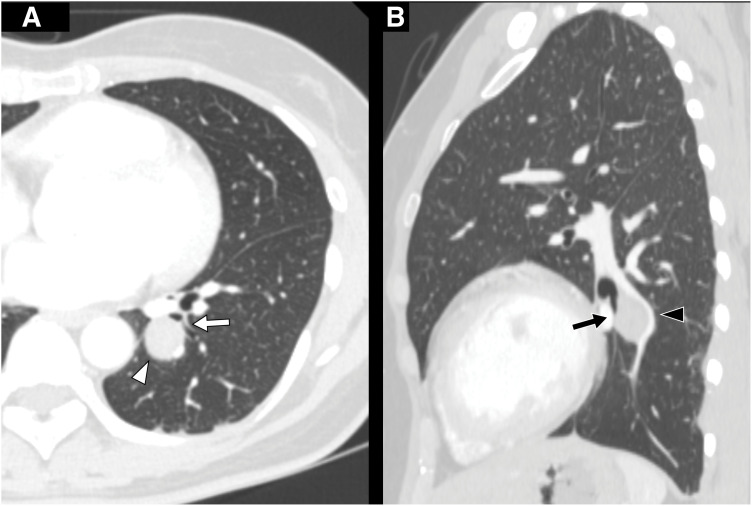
Chest CT images. (A) Axial CT showing a well-circumscribed 2.4 cm nodule in left S10 (white arrowhead), adjacent to the central portion of bronchus B10 (white arrow). (B) Sagittal CT demonstrating compression of pulmonary artery A10 (black arrowhead) and stenosis of bronchus B10 (black arrow) caused by the tumor. S10, segment 10
